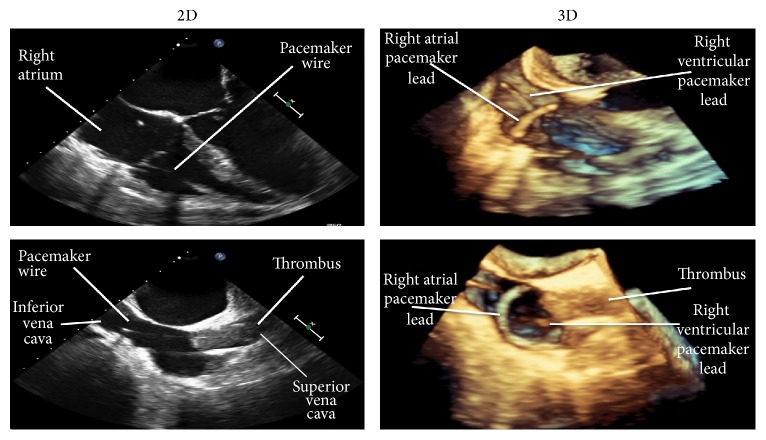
3D echocardiogram demonstrated the tract of the pacemaker wires clearly and showed that the venous thrombosis was separate from the pacemaker wires. In comparison, 2D echocardiography is less distinct.
